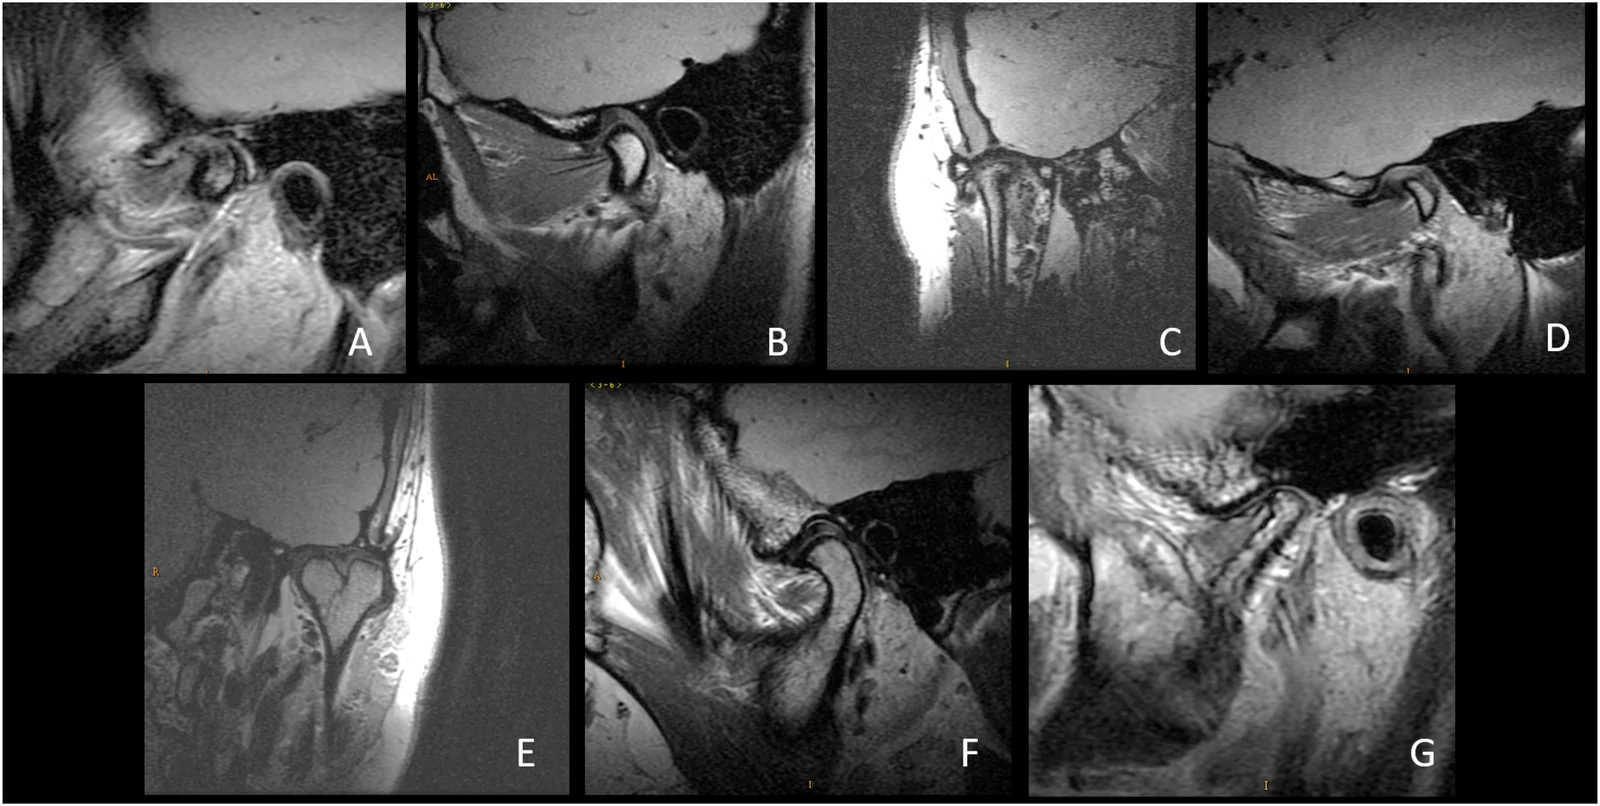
MRI images of the TMJ showing examples of the assessed condylar alterations: A - erosion; B - condylar cortical thickening; C - limited condyle width; D - limited condyle thickness; E - presence of bifid condyle/rudimentary bifidity; F - condylar hyperplasia; G - condylar hypoplasia.
